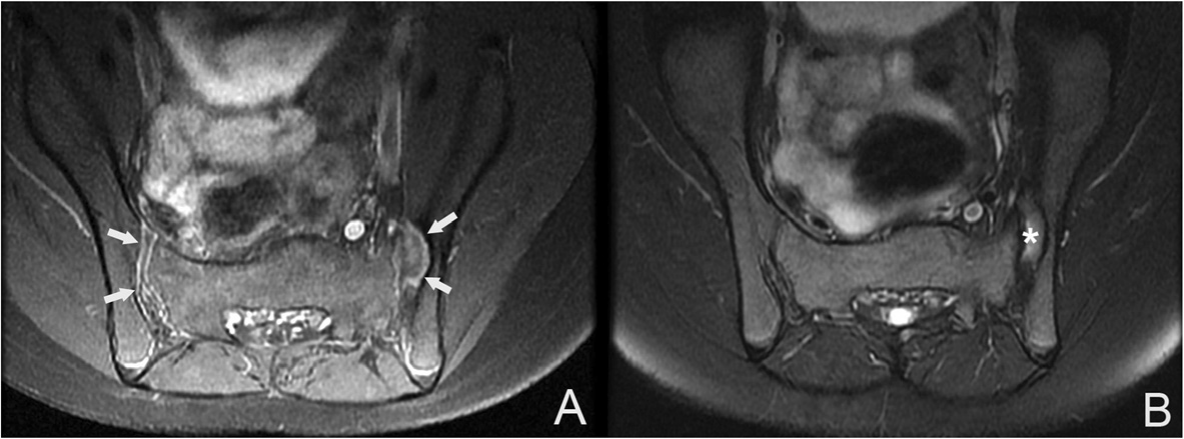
Sacroiliitis without bone marrow edema. Axial MRI images of the sacroiliac joints in an 8 year-old female with spondyloarthropathy shows bilateral synovial enhancement (arrows) and a large erosion in the left joint on the T1-weighted gadolinium contrast enhanced image (A). Although the synovium in the erosion contains increased signal intensity (asterisk), no surrounding bone marrow edema is visible about either sacroiliac joint on the T2-weighted fat-suppressed image (B).
